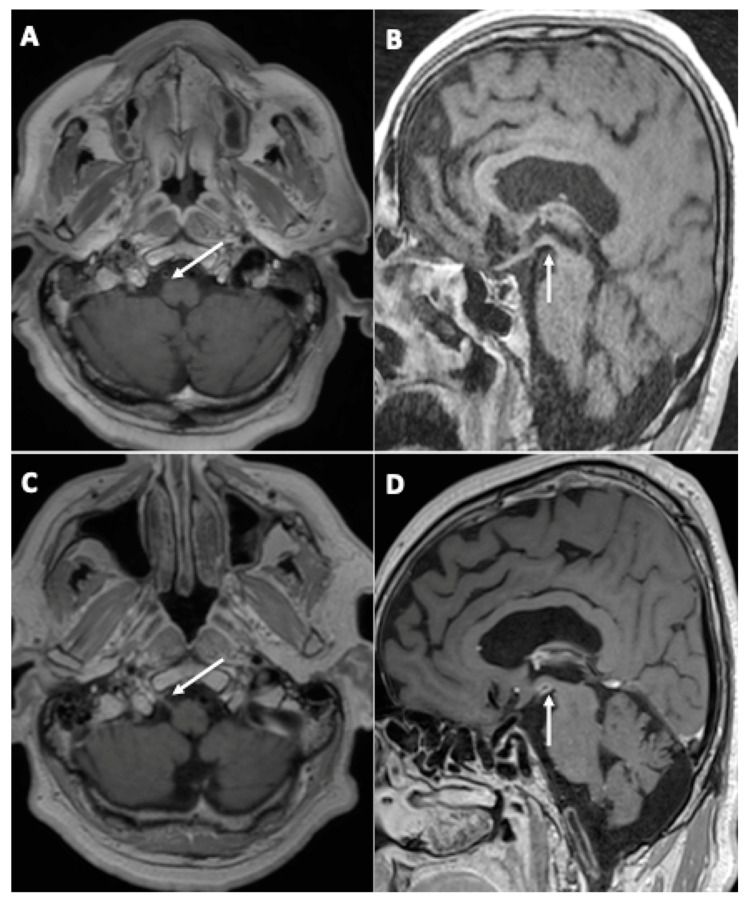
A 67-year-old man (#20) (A,C) and a 61-year-old man (#7) (B,D), both initially hospitalized (ICU), who each underwent a second MRI during follow-up 112 (A,C) and 99 (B,D) days later, respectively. Axial (A,C) and sagittal (B,D) post-contrast three-dimensional T1 weighted spin-echo MR imaging. Appearance of contrast enhancement affecting the right hypoglossal nerve (C) (neuritis), and the wall of the posterior cerebral arteries suggestive of cerebral vasculitis.
